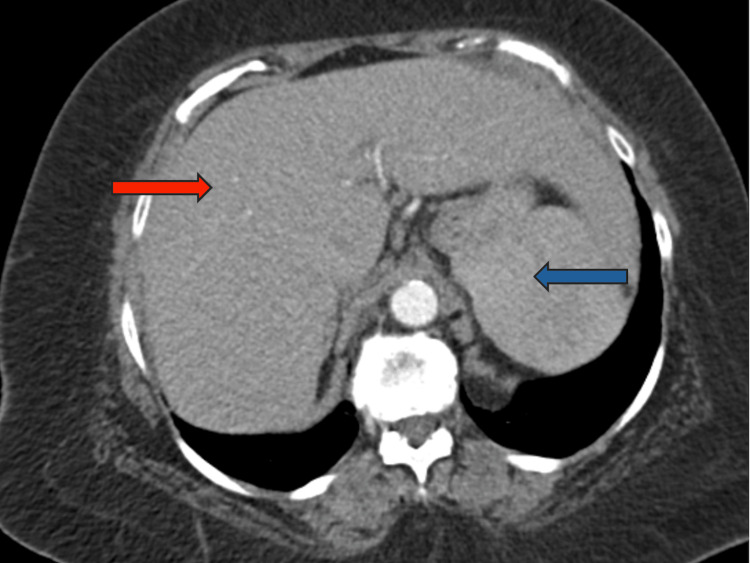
Axial CT scan of the abdomen showing mild cirrhosis (red arrow) and splenomegaly (blue arrow)
